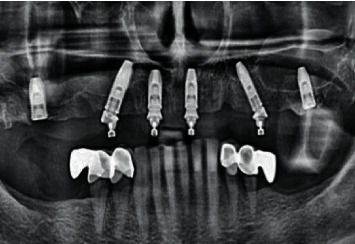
Radiographic control of implant integration (8 weeks).
